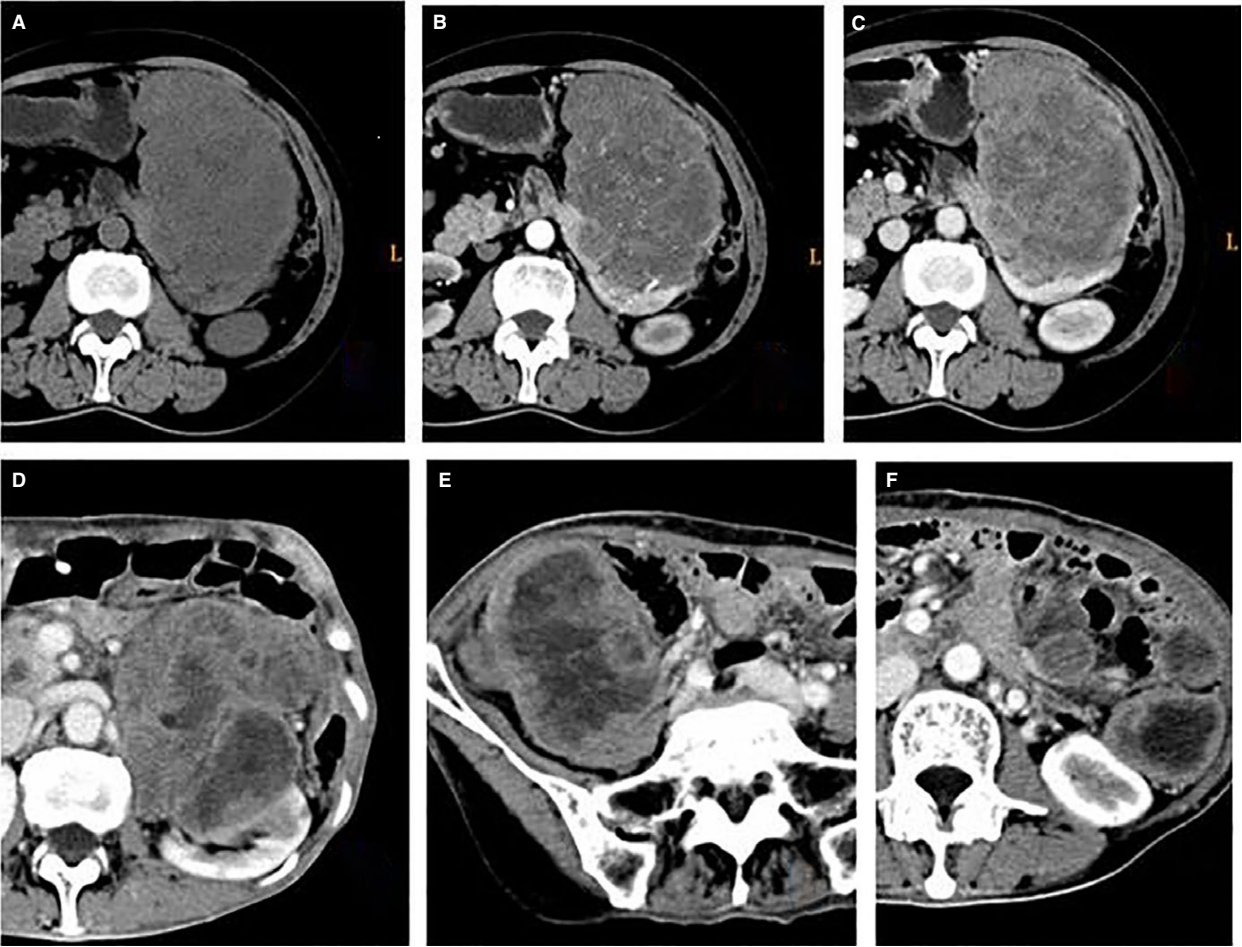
A 67‐y‐old woman with a exophytic GIST in the stomach, high risk. Unenhanced and enhanced CT (A‐C) shows a large, irregular, ill‐defined mass with necrosis and heterogeneous enhancement. Nine months after resection, enhanced CT (D‐F) demonstrates the multiple intraperitoneal recurrence and metastases
